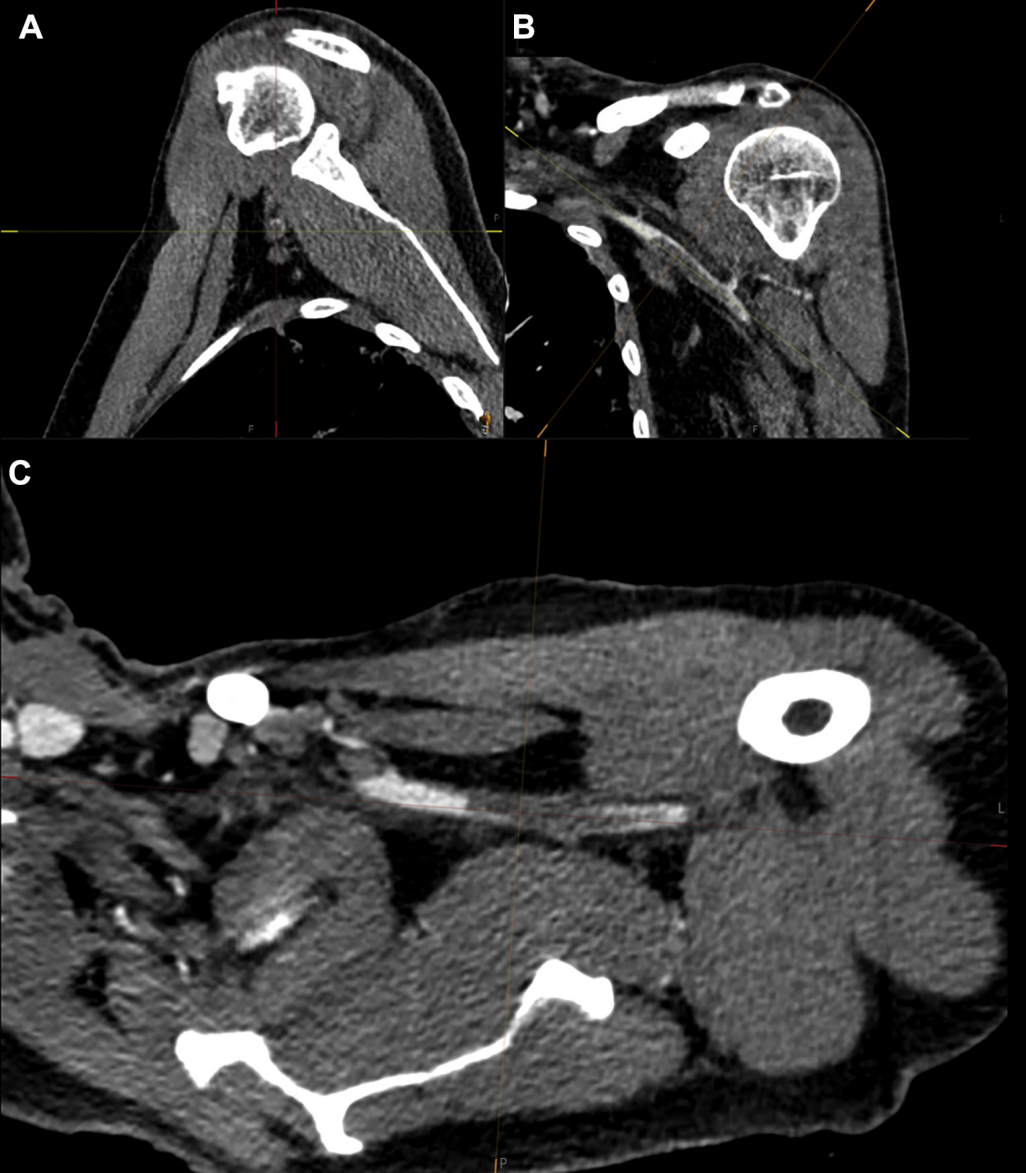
A 55-year-old COVID-19-positive man with a medical history of diabetes with oral metformin use presented with a pale, pulseless left hand without muscle weakness and minimal sensory loss of the fingers. Acquired computed tomography angiography (CTA) imaging showed subclavian artery occlusion. A, Sagittal view. B, Coronal view. C, Axial view.
